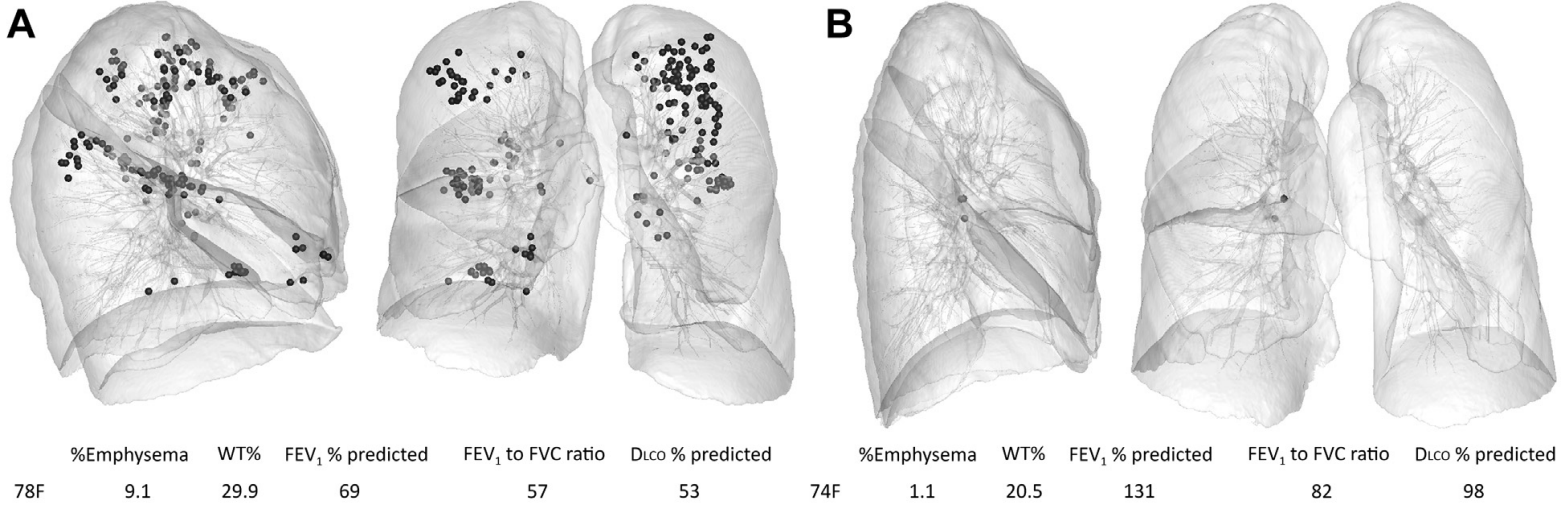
Images of representative patients with high and low scores of WT% and %Emphysema: dark spheres indicate quantitative CT imaging-based emphysematous regions. Dlco = diffusing capacity of the lungs for carbon monoxide; %Emphysema = percentage of emphysema; WT% = wall thickness percentage.
